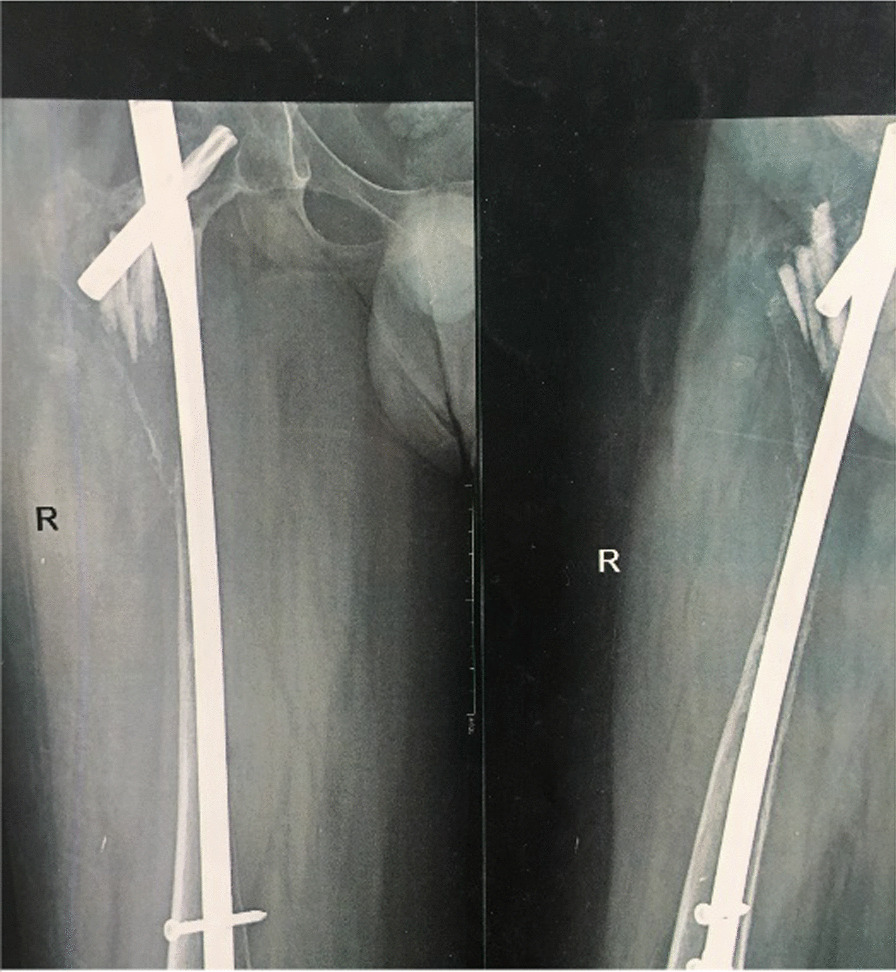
Right femur Anterior Posterior (AP) and lateral X-ray; note allograft resorption and bone disappearing
